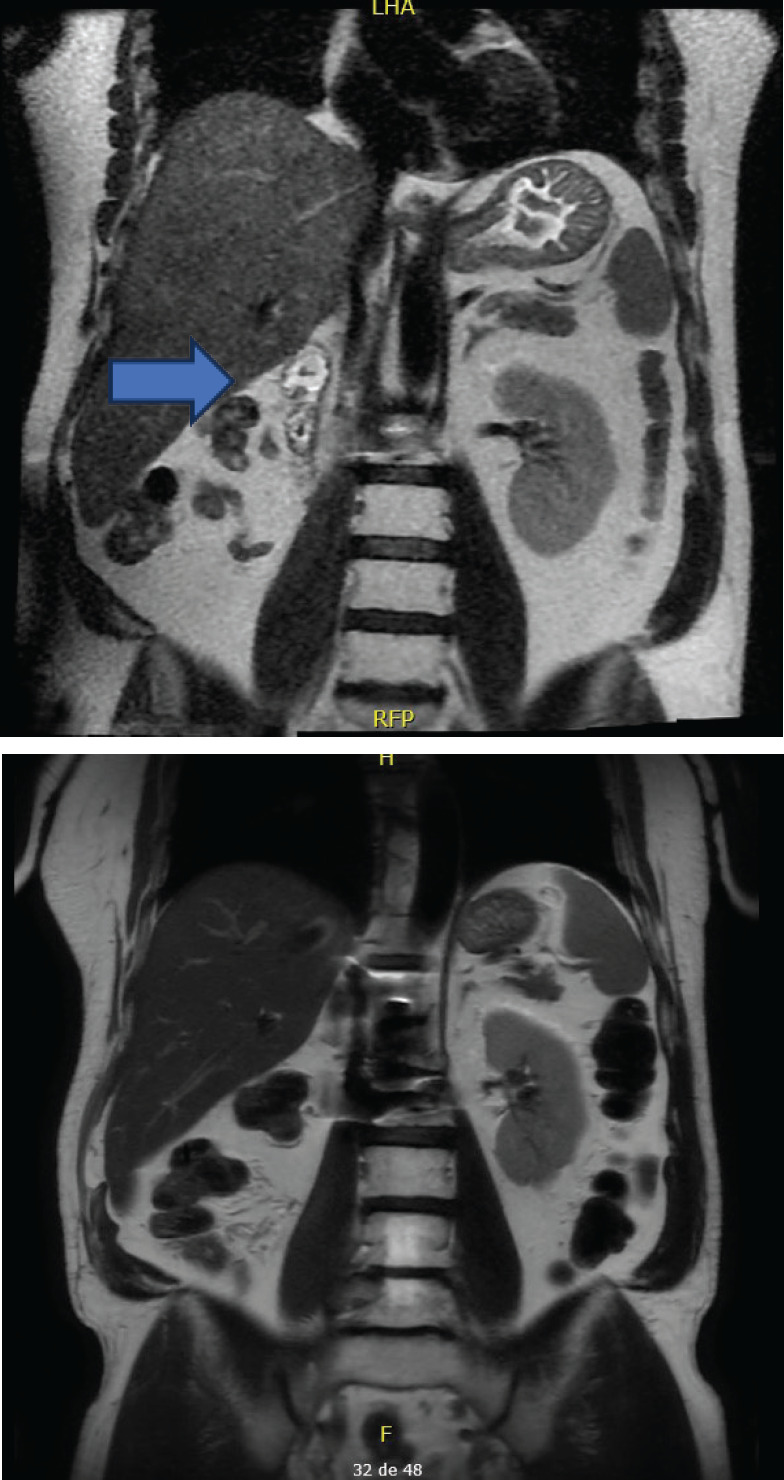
Evolution of the patient’s MRI over different time periods. Left: Abdominal MRI at the beginning of immunomodulatory therapy (30 December 2016) – showing post-right nephrectomy and two small solid nodules with neoplastic appearance found in the right renal fossa. Right: Abdominal MRI after 50 cycles of immunomodulatory therapy (8 July 2022) – showing a complete response with no secondary neoplastic involvement observed in the abdomen and pelvis.
